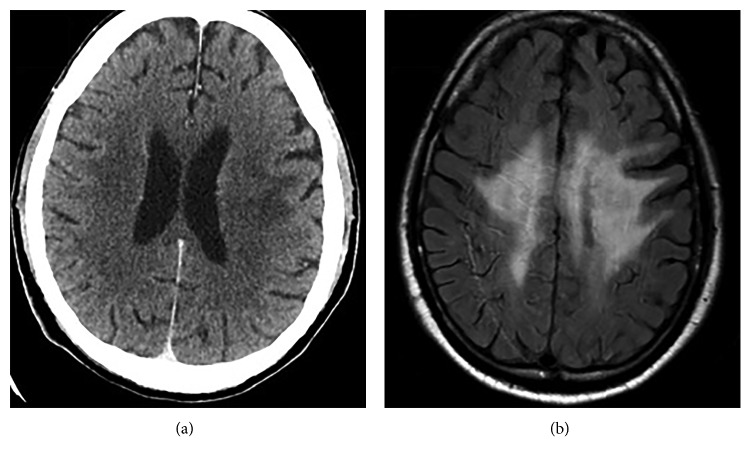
(a) Computed tomographic scan showed a focal lesion in the white matter of the left cerebral hemisphere. (b) FLAIR magnetic resonance imaging sequences showing lesion with surrounding cerebral edema that involved posterior central cerebral convolution, posterior limb of internal capsule, corpus callosum of the left hemisphere, and right central cerebral convolution.
